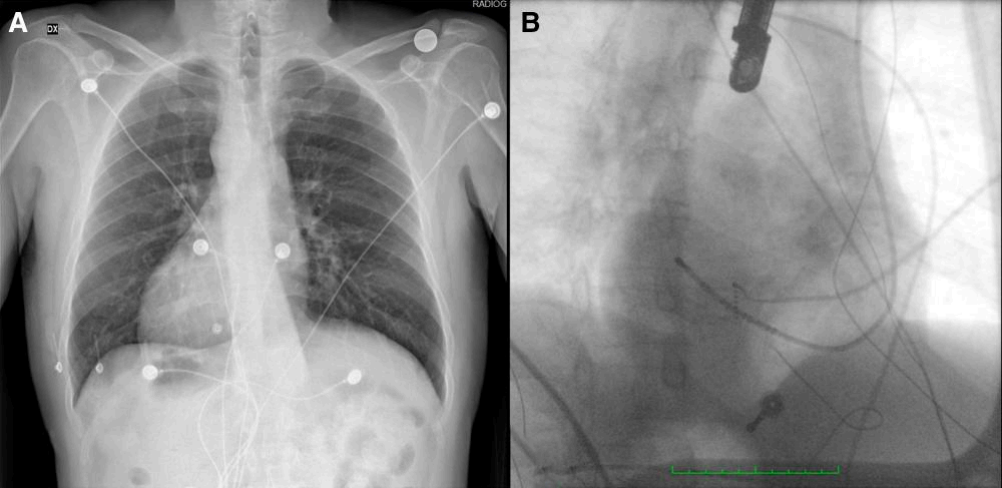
(*A*) Standard chest radiography highlighting dextrocardia. (*B*) Fluoroscopy right anterior oblique (RAO) projection with decapolar catheter inserted in the coronary sinus mimicking left anterior oblique (LAO) projection.
